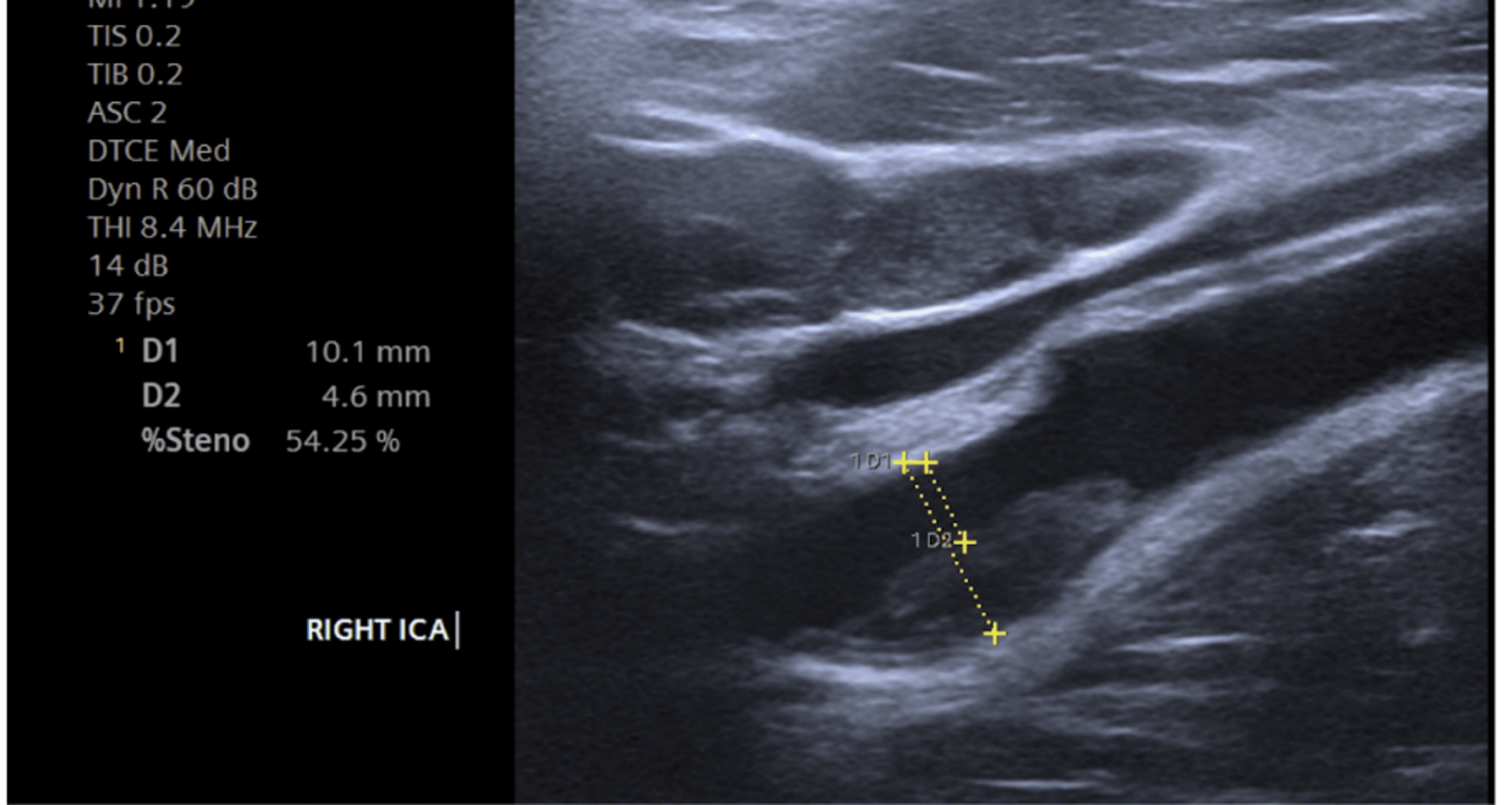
Carotid artery doppler ultrasound Revealed a homogeneous soft plaque at the proximal right internal carotid artery with 50% stenosis.
